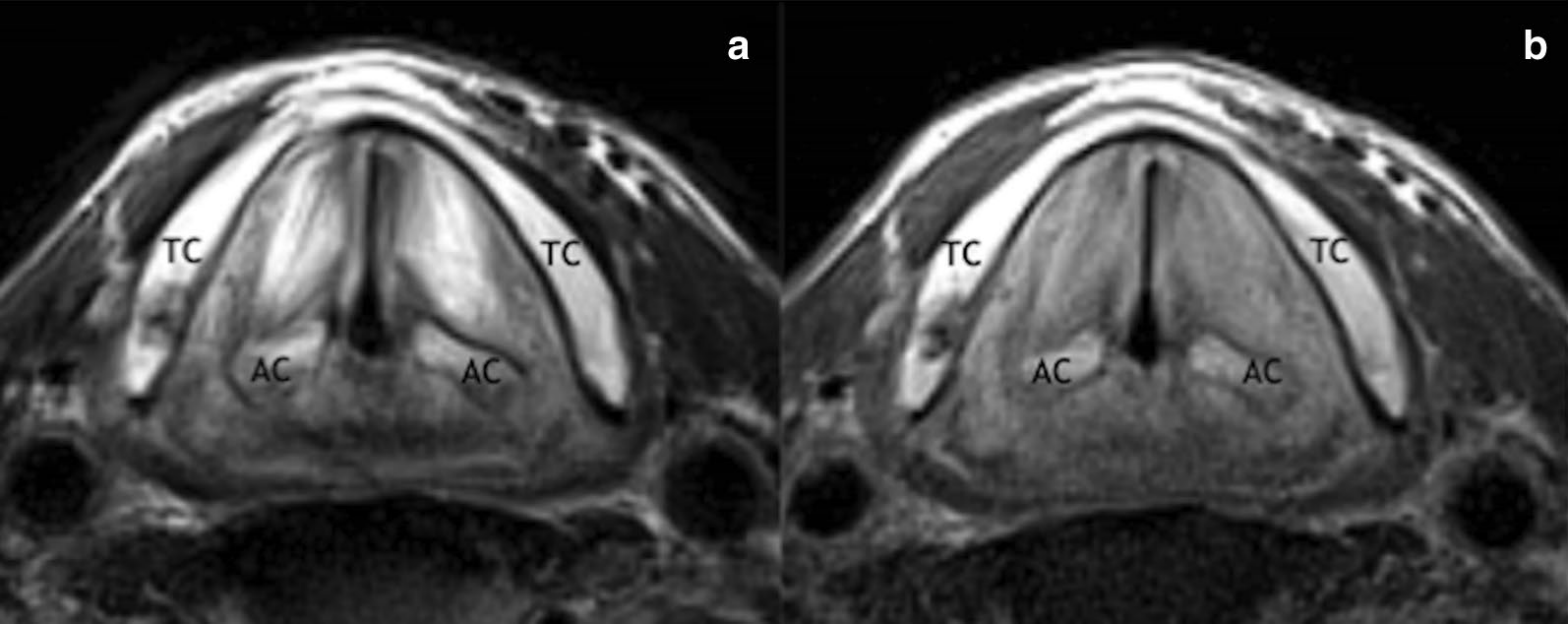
Chronic edema of the true vocal folds 14 years after ChT/RT in a 72-year-old man with lymph node metastasis from unknown primary. Axial T2-weighted (a) and T1-weighted (b) images acquired with surface-coil MRI of the larynx show diffuse T2-hyperintensity and slightly T1-hyperintensity of the true vocal folds. TC, thyroid cartilage; AC, arytenoid cartilage
